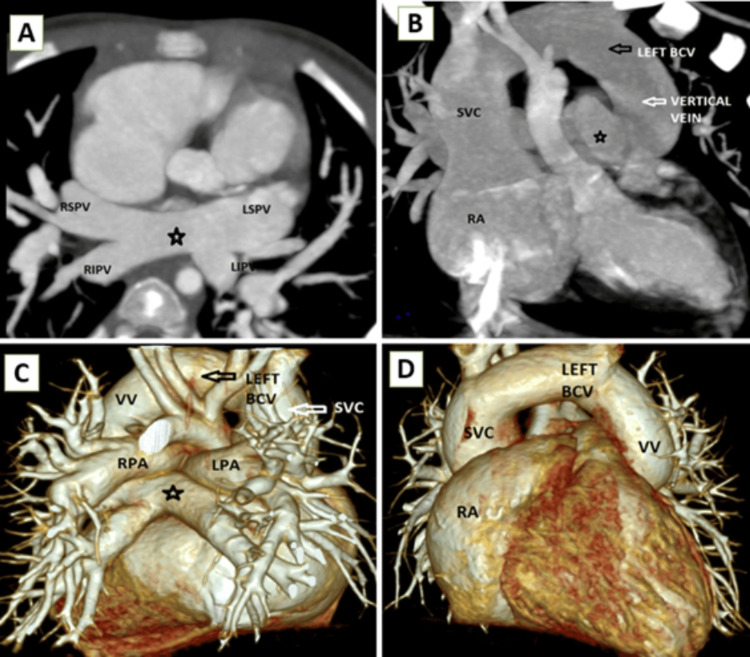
Supracardiac TAPVC (A) cardiac CT axial MIP sections showing confluence (black star) of all four pulmonary veins, (B) CT coronal post-contrast MIP section showing confluence of all four pulmonary veins (black star) joining to form a vertical vein draining into the SVC through the left brachiocephalic vein (LEFT BCV), and (C and D) 3D VR CT reconstructed images viewed from the posterior aspect (C) and anterior aspect (D) demonstrating anomalous drainage of all pulmonary veins into a dilated VV and SVC indirectly to the RA TAPVC: total anomalous pulmonary venous connection, MIP: maximum intensity projection, CT: computed tomography, SVC: superior vena cava, VR: volume rendered, VV: vertical vein, RA: right atrium, RSPV: right superior pulmonary vein, RIPV: right inferior pulmonary vein, LSPV: left superior pulmonary vein, LIPV: left inferior pulmonary vein, LEFT BCV: left brachiocephalic vein
